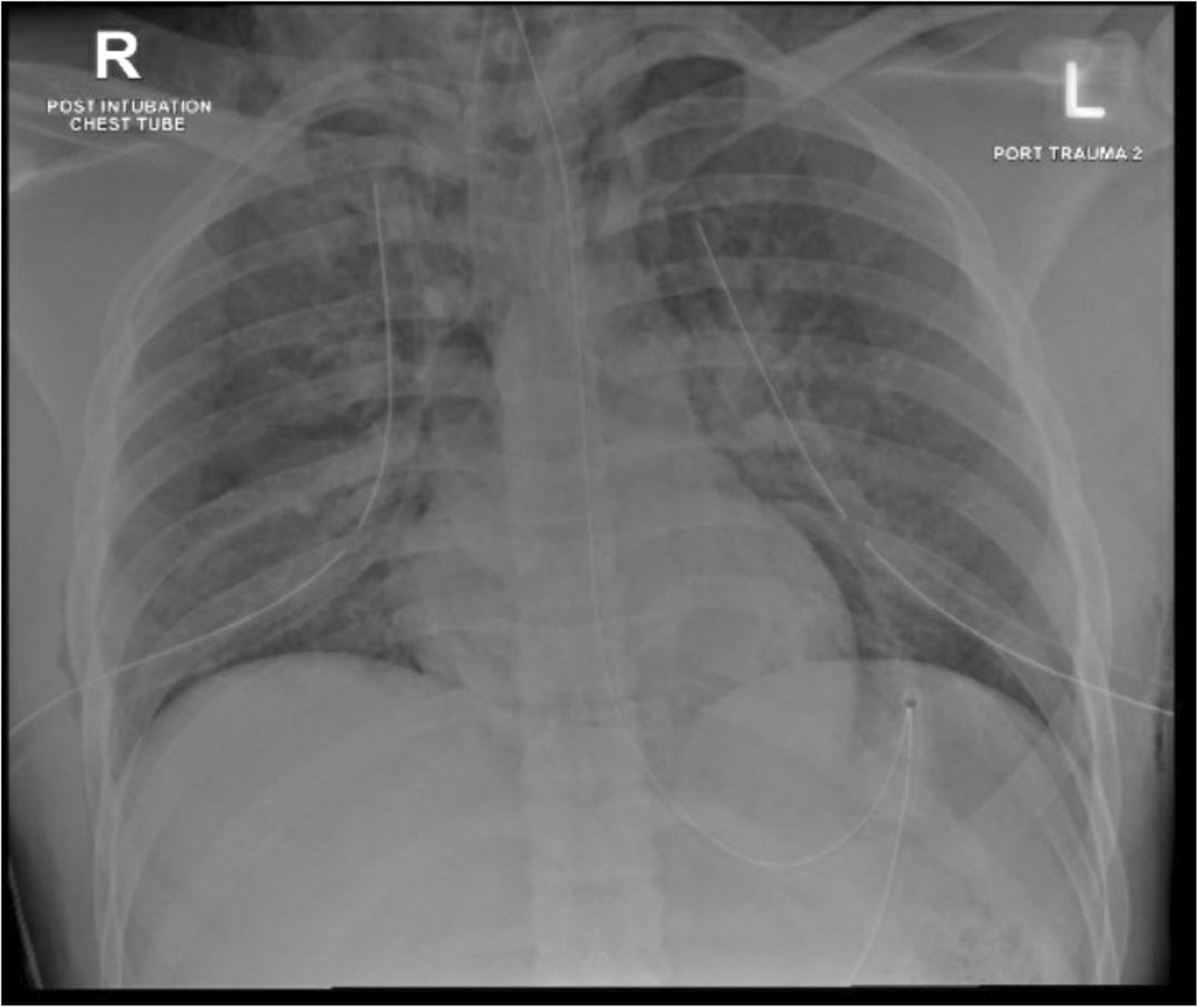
Chest radiograph after tracheal intubation and insertion of bilateral chest drains.
